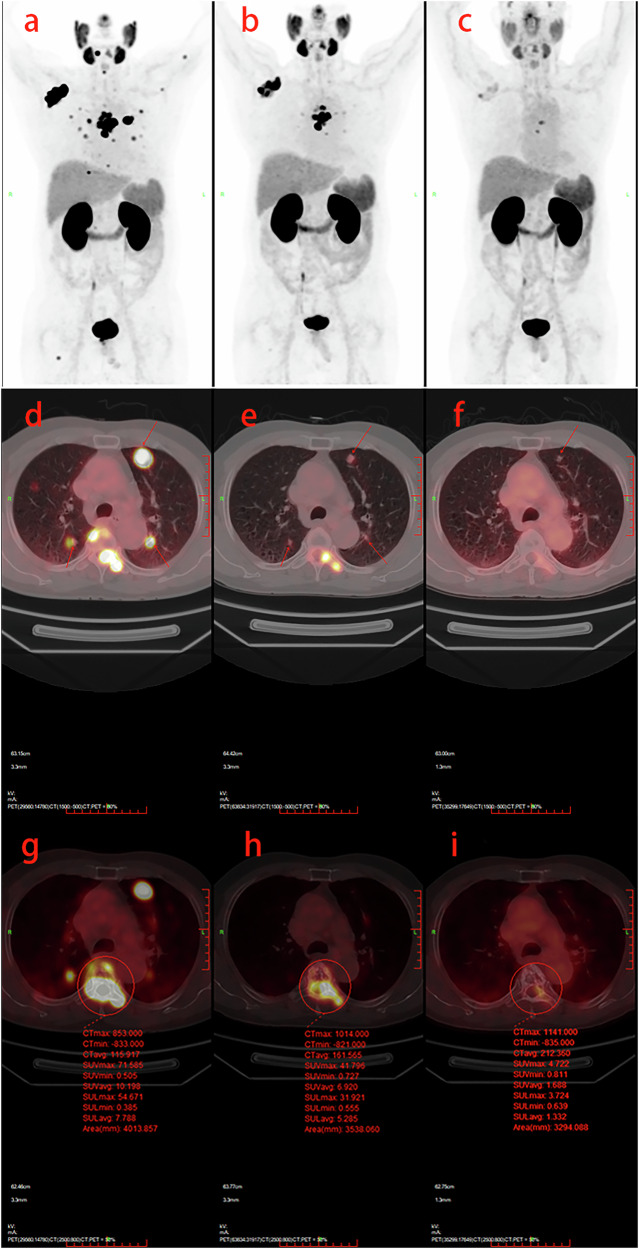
Representative ^18^F-PSMA-1007 PET/CT imaging demonstrating treatment response to ^225^Ac-PSMA-CY313 therapy. Images show from a representative patient at baseline (a, d, g), after one cycle (b, e, h), and after four cycles (c, f, i) of ^225^Ac-PSMA-CY313 treatment. The area indicated by the red arrow and within the red circle represents the lesion expressing PSMA
